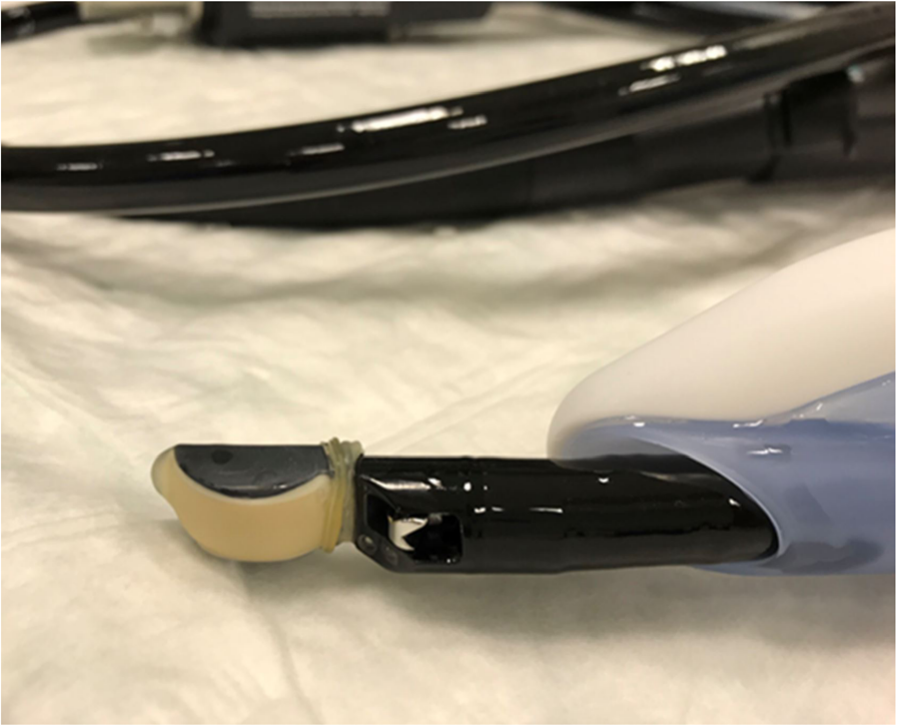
Endoscopic ultrasound probe passing through the LMA Gastro™
